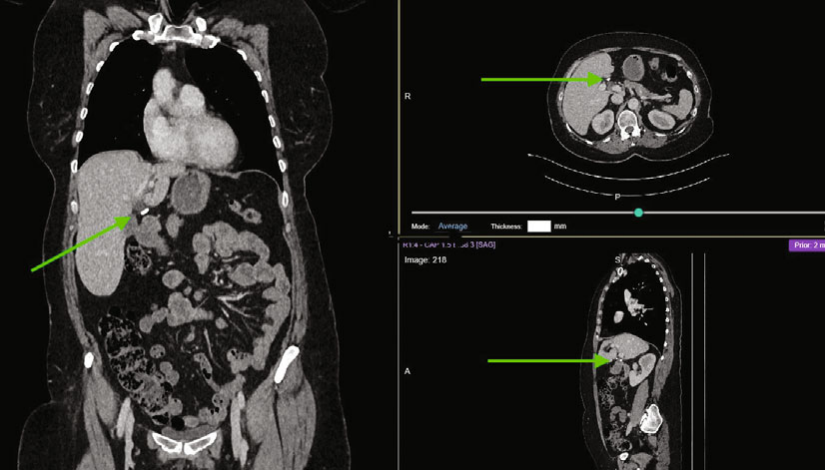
Preoperative CT TAP scanning showing radio opaque lesion in the upper abdomen suspicious for cancer recurrence.
